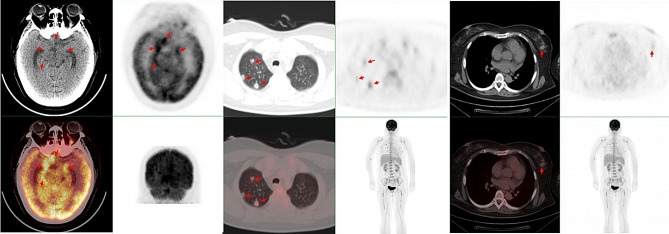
PET/CT findings. Multiple hypermetabolic foci are present in the brain parenchyma, suggestive of infectious or inflammatory processes. Bilateral pulmonary nodules also demonstrate increased FDG uptake, consistent with probable infectious etiology. The study further reveals metabolic activity in the paranasal sinuses, compatible with sinusitis. A left breast nodule shows low-grade FDG avidity, likely benign without features indicating malignancy
